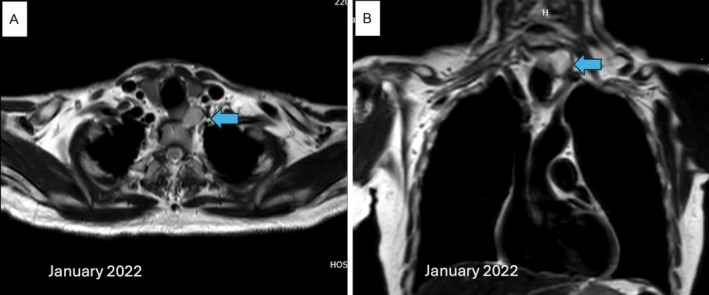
Preoperative MRI from January 2022 showing a thin‐walled cyst in the left paravertebral region at C7–T1 (arrows). (A) Axial and (B) Coronal planes.
